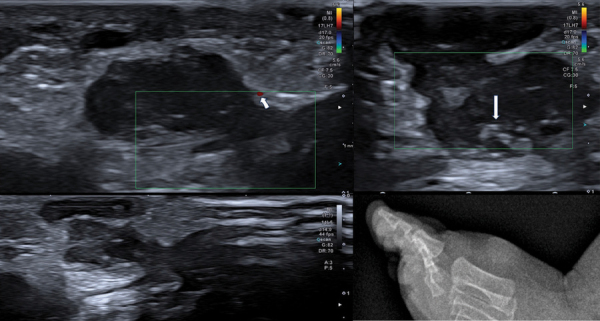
US images of the lesion showing little surrounding vascularization (arrow top left) and small calcifications (arrow top right). X-ray of the lesion (bottom right).
